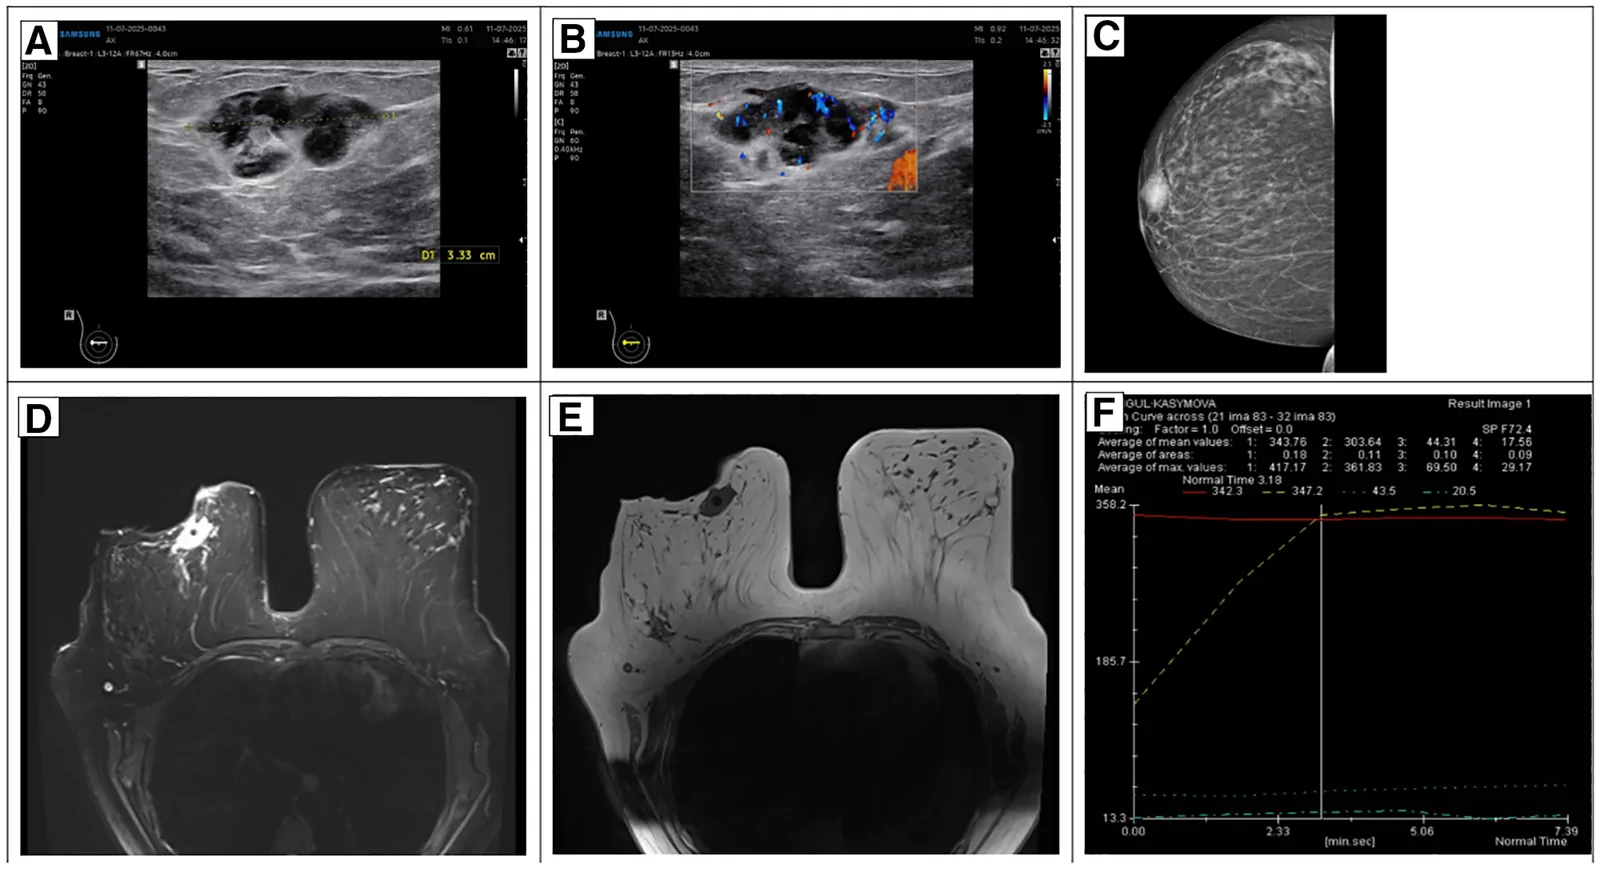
Imaging findings of primary breast diffuse large B-cell lymphoma in this patient. (A) Breast ultrasonography showed a hypoechoic mass with an ill-defined margin, an irregular shape, and heterogeneous internal echogenicity. (B) Color Doppler Flow Imaging demonstrated peripheral vascular signals around the lesion. (C) Mammography showed a high-density lesion with an ill-defined margin. (D, E) Breast magnetic resonance imaging demonstrated a lobulated mass measuring 24 × 29 mm in the upper-outer quadrant of the right breast. The lesion showed slightly prolonged T1- and T2-weighted signal intensity. (F) The time–signal intensity curve showed a plateau pattern, suggesting a possible malignant lesion.
